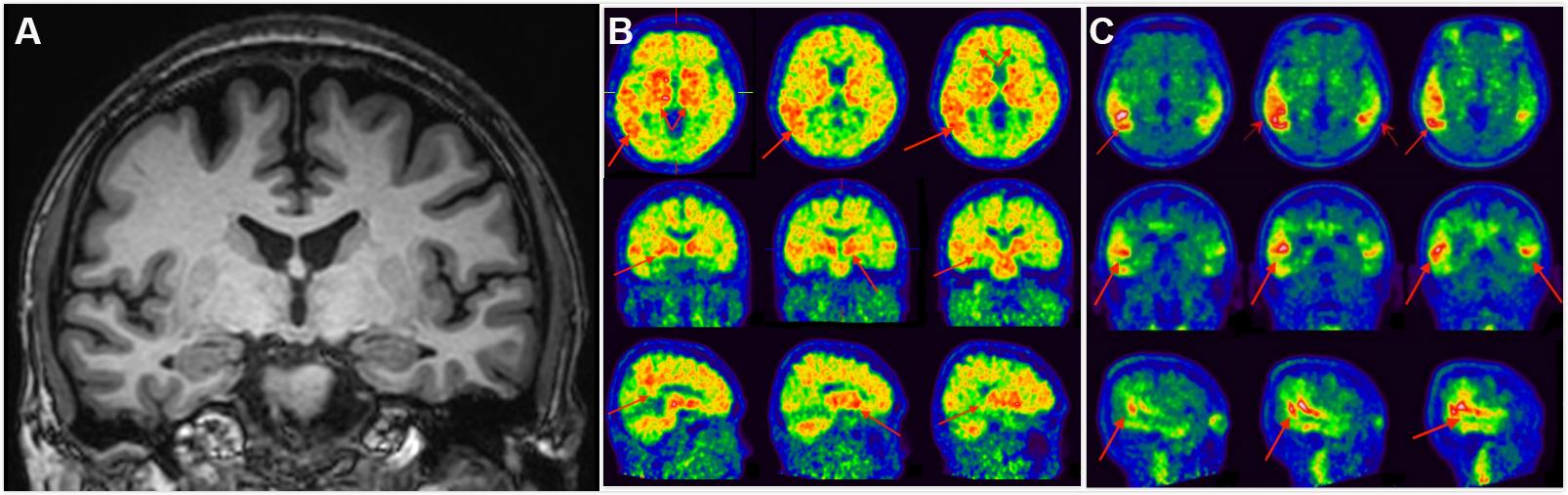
Clinical utility of AD-RAI in MCI subjects. A 50-year-old man with 16-year education had complaints of impaired short-term memory for 4 years. Z-score in Trial 4 of HKLLT was -2.97 SD (≤ -1 SD, i.e. MCI). The average visual MTA rating score was 0 suggestive of non-AD (A). HV measures also suggested non-AD, with a normal raw HV of 7.25mL (> 6.07mL) and HF of 0.48% (> 0.41%). However, AD-RAI was 0.68 (> 0.5) suggestive of AD. Subsequent PIB PET (B) and T807 PET (C) confirmed PIB and T807 retention, respectively (red arrows). The final diagnosis of this subject was prodromal AD. Abbreviations: AD-RAI=Alzheimer’s disease resemblance atrophy index; MCI=mild cognitive impairment; MTA=medial temporal lobe atrophy; HKLLT=Hong Kong List Learning Test; SD=standard deviation; MRI=magnetic resonance imaging; HV=hippocampal volume; HF=hippocampus fraction; PET=positron emission tomography; SVD=small vessel disease.
